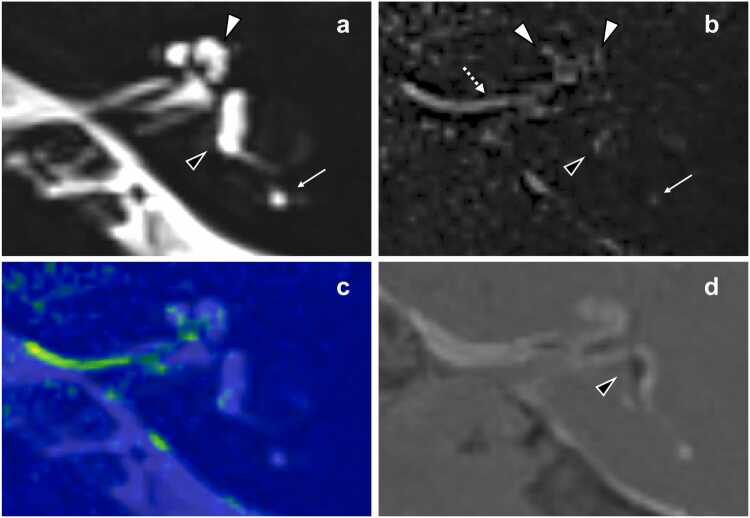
50-year-old female with inner ear high signal and endolymphatic hydrops, associated with bilateral auditory symptoms (hearing loss, tinnitus, and ear fullness). a MRC: Hyperintensity is observed within lymph fluid located in the left cochlea (white arrowhead), vestibule (black arrowhead) and posterior semicircular canal (arrow). b Non-contrast HT2-FLAIR imaging: Abnormal signals are depicted in the left cochlea (white arrowheads), vestibule (black arrowhead) and posterior semicircular canal (arrow). The vestibular high signal, located peripherally, seems to be within the perilymphatic space. Notably, linear hyperintensity is observed within the left internal auditory canal (dotted arrow), indicative of dural/subdural abnormality. c Fusion image generated from MRC and non-contrast HT2-FLAIR image. d 4-hour delayed contrast-enhanced HYDROPS: The left vestibule shows significant hydrops (black arrowhead), while the cochlea demonstrates no evidence of hydrops. Abbreviations: MRC *MR cisternography*, HT2-FLAIR *heavily T2‑weighted three-dimensional fluid attenuated inversion recovery*, HYDROPS *hybrid of reversed image of positive endolymph signal and native image of positive perilymph signal*.
